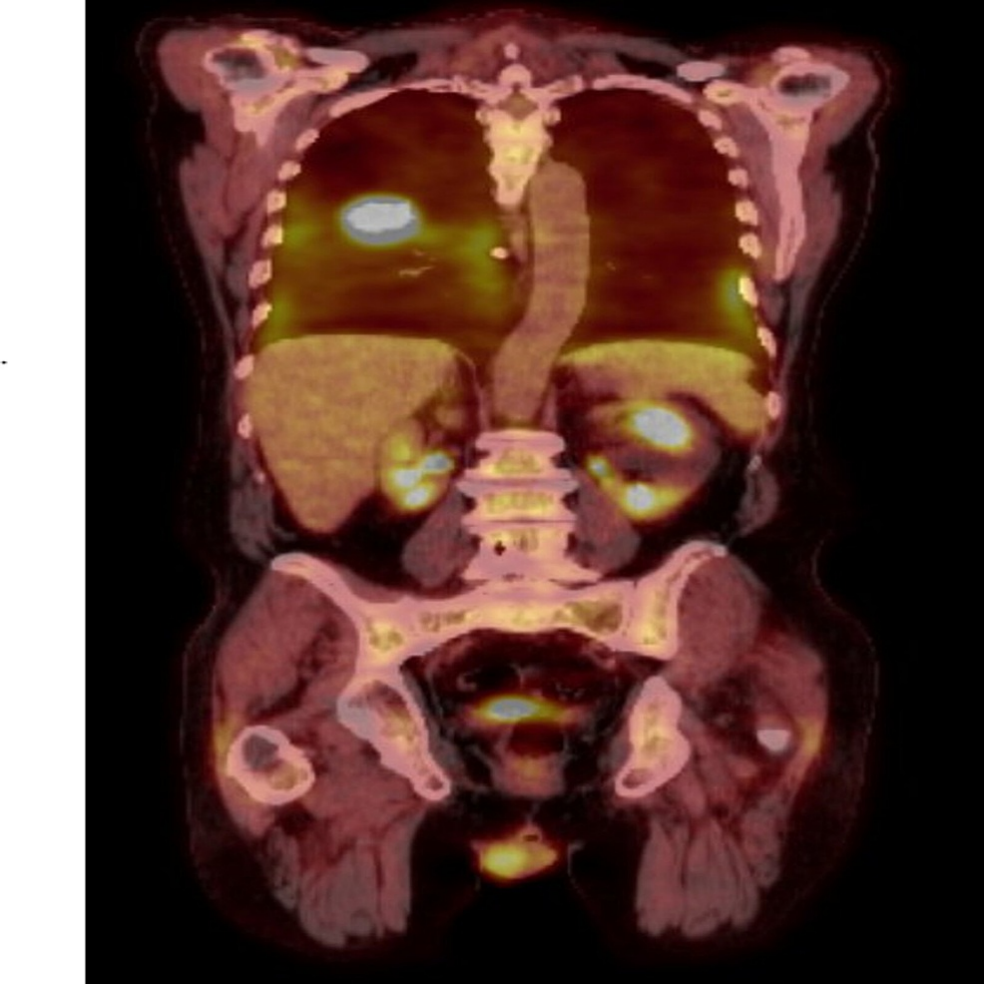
PET-CT scan showing a right perihilar mass with hilar lymphadenopathy. PET-CT: positron emission tomography-computed tomography
